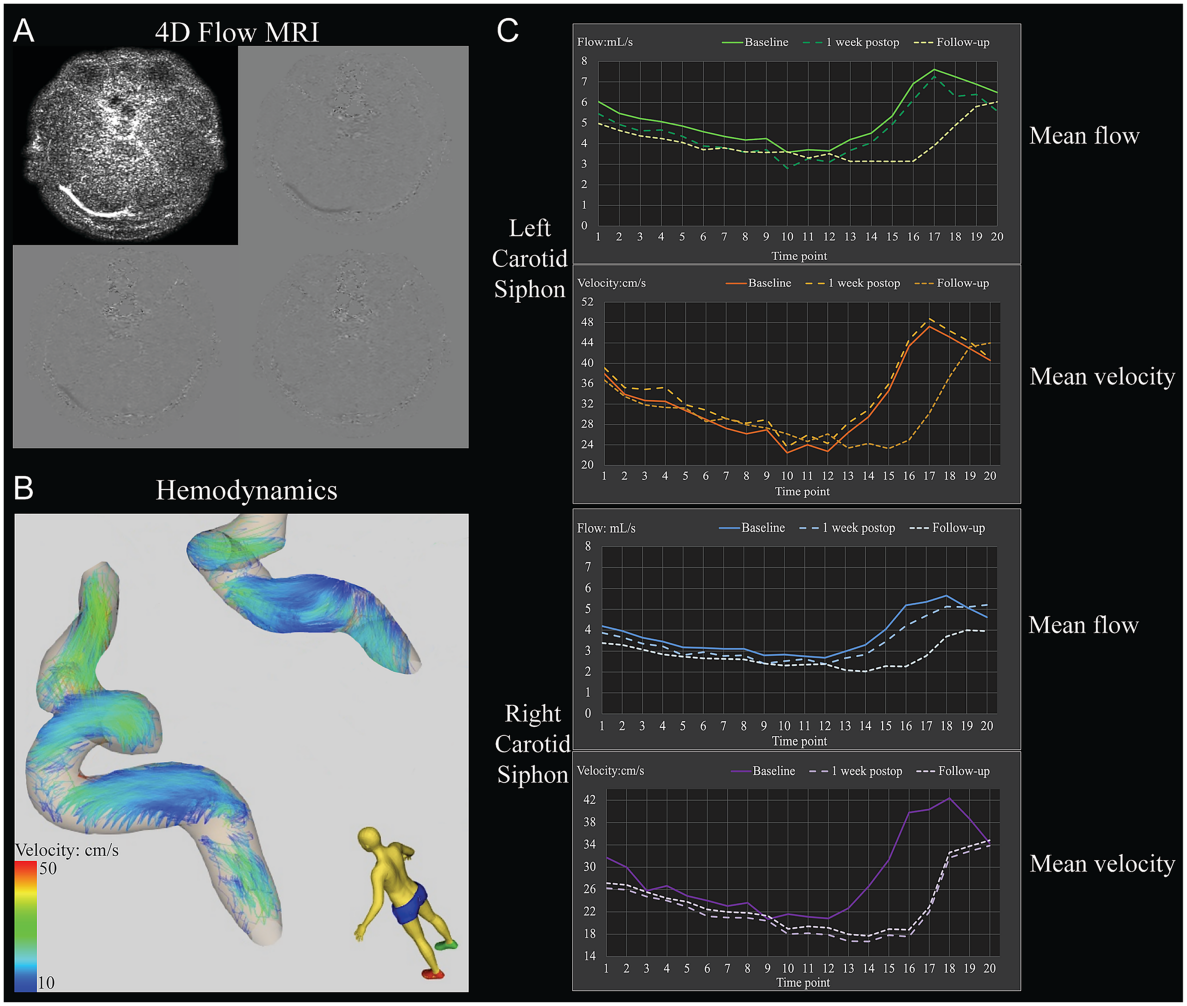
Example of the determination of hemodynamic parameters in the carotid siphons of a 31-year-old woman with moyamoya angiopathy who underwent left-side revascularization. (A) Raw 4D Flow MRI. (B) Pathline visualization of the bilateral carotid siphons. (C) Hemodynamic parameters included mean flow and mean velocity through the bilateral carotid siphons at different time points within one cardiac cycle before revascularization (baseline), 1 week after surgery, or 1 year after surgery (follow-up).
